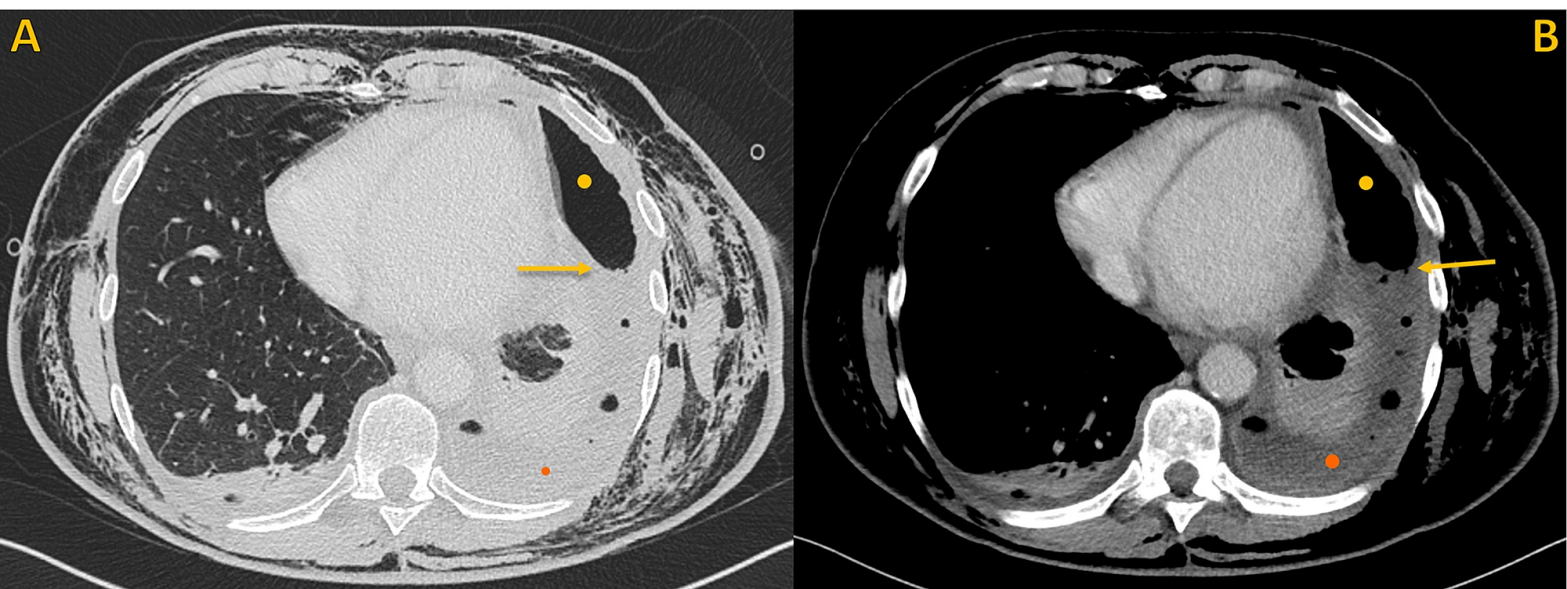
Non-contrast chest CT, same axial plane Non-contrast chest CT, axial images. A) Lung window and B) Soft tissue window, demonstrates reduction of the left pneumothorax (yellow circle) and the air-fluid level of the hydropneumothorax (yellow arrow). Note left pleural effusion (orange circle), with multiple air bubbles inside.
